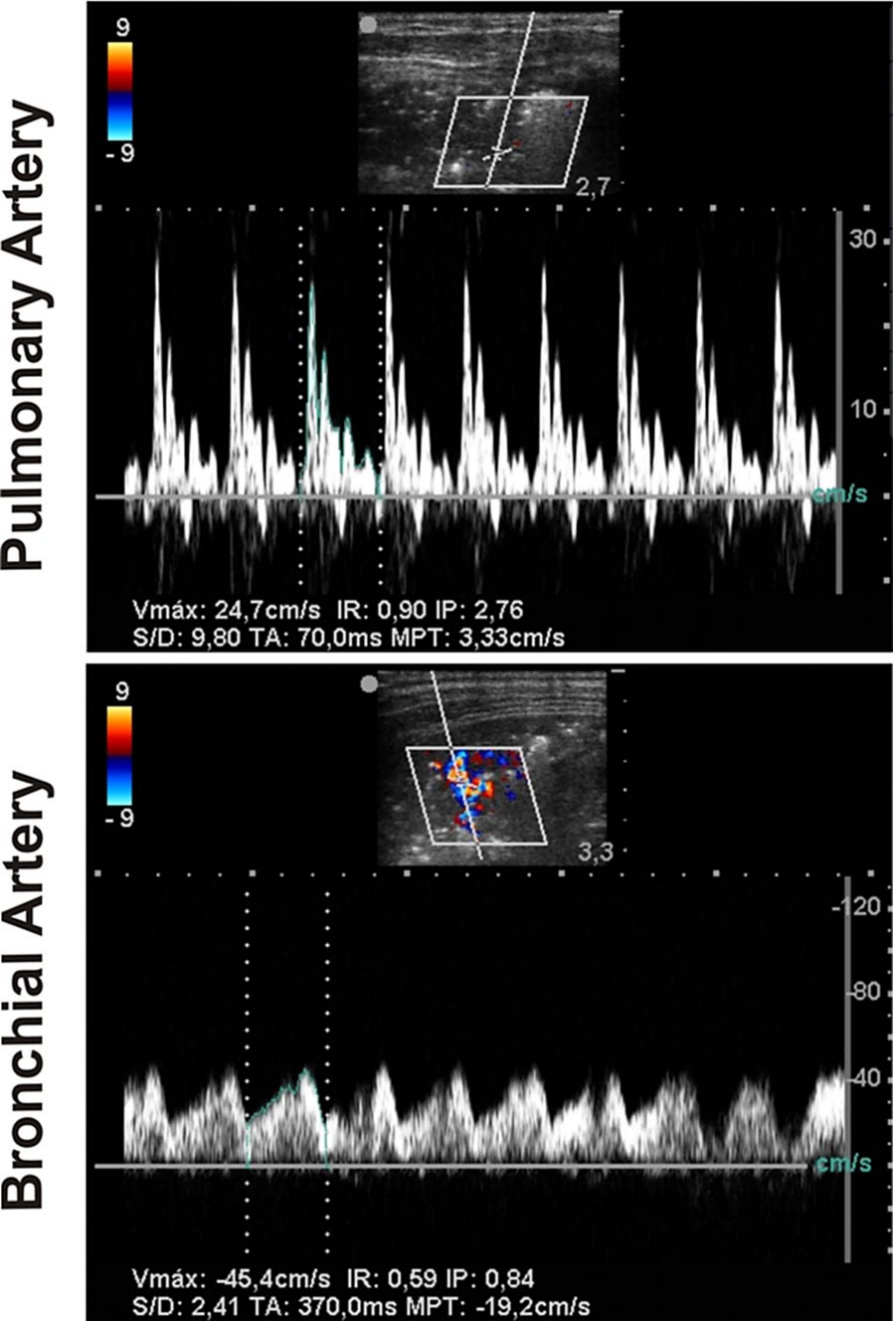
Spectral Doppler analysis of the three types of vessels within the lung. Spectral Doppler analysis of a pulmonary artery (*upper*) and a bronchial artery (*lower*) within a representative anesthesia-induced atelectasis in 4-year-old patient. Spectral Doppler was performed during a brief expiratory pause. Pulmonary artery showed lower Doppler maximal velocity and higher resistance (IR) and pulsatile (IP) indexes than the bronchial artery presumably due to hypoxic pulmonary vasoconstriction
